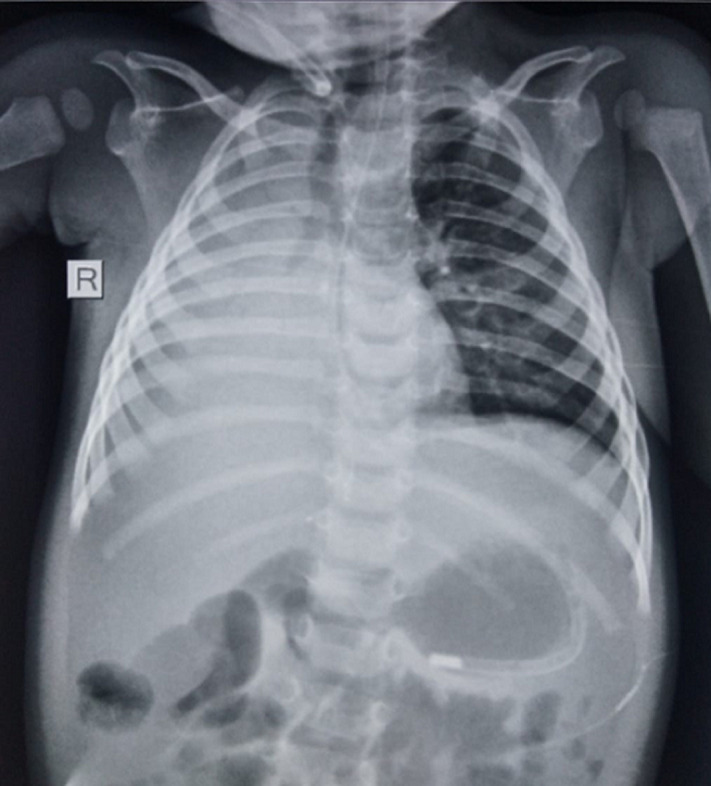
chest X-ray showing right opaque hemithorax with trachea pulled to the right side with nasogastric tube in situ
